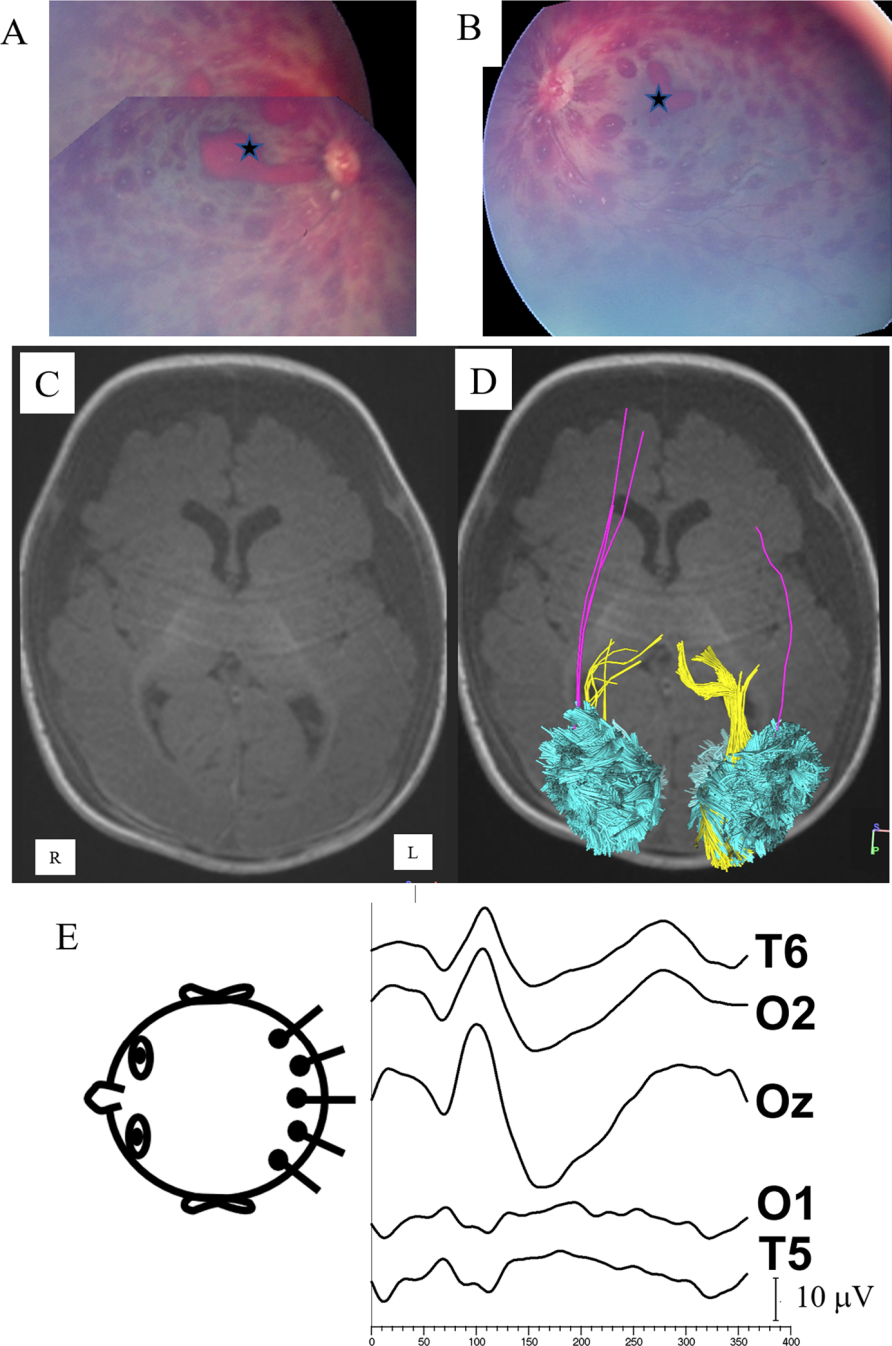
A child presenting at 151 days age with acute respiratory failure, status epilepticus, acute on chronic subdural hematomas, skull fracture, and leg fractures. RetCam imaging of the right eye A and left eye B show numerous pre- and intra-retinal hemorrhages with white centers, hemorrhage within both macula (stars), diffuse macular edema with sub-hyaloid hemorrhages. An MRI at 152 days age showed diffusion restriction on neuroimaging with areas of edema. C Axial T1-weighted image, D same image with reconstructed tracts superimposed showing reduced volume of the right optic radiations (yellow), bilateral reduced volume of the inferior fronto-occipital fasciculus (purple), and mild volume loss of the vertical occipital fasciculus. (cyan). E, VEP at 0.5 years age showed a larger response over the right hemisphere (paradoxical lateralization). At last follow-up visual acuity was 0.07 logMAR and he had seizures, mild developmental delay, and spastic left hemiparesis
